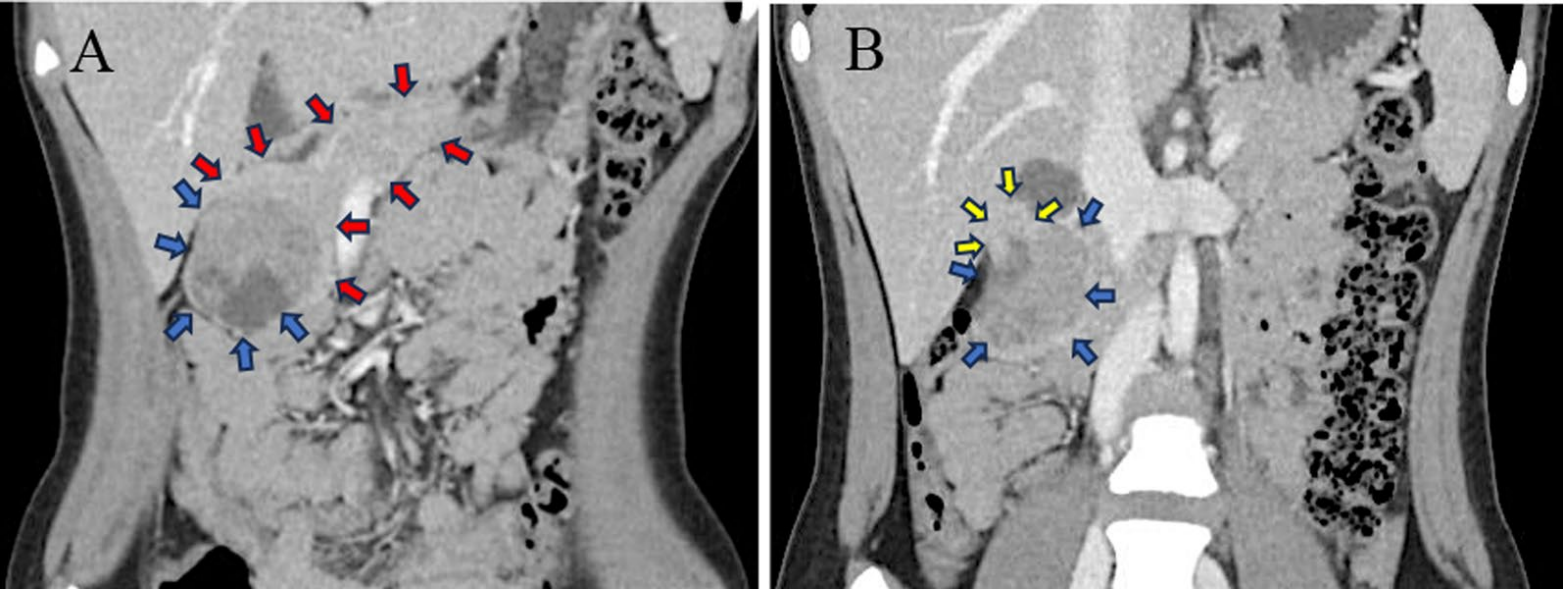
Images of preoperative MRI. Tumor (blue arrow) and pancreas (red arrow) (A). Tumor (blue arrow) and duodenum (yellow arrow) (B)
